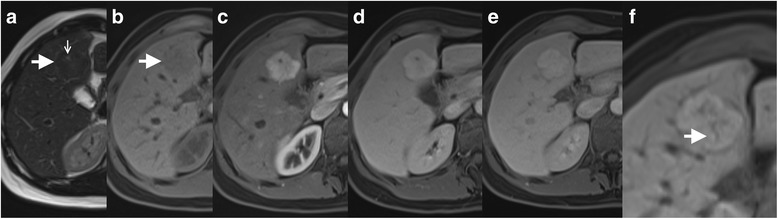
MRI examination of a 33-year-old female; all the images are in the axial plane. Fig. a and b present slightly hypointense FNH in segment IV of the liver (arrow) on T2- and T1-weighted images. Central scar (small arrow) is hyperintense on T2-weighted images (Fig a). In hepatic arterial phase, the lobulated lesion presents strong enhancement (Fig. c). In portal venous phase (Fig. d) and equilibrium phase (Fig. e) the lesion stays hyperintense, however, the enhancement is weaker than in hepatic arterial phase. In hepatobiliary phase (Fig. f) the lesion is hyperintense and enhancement of the scar is visible (arrow)
